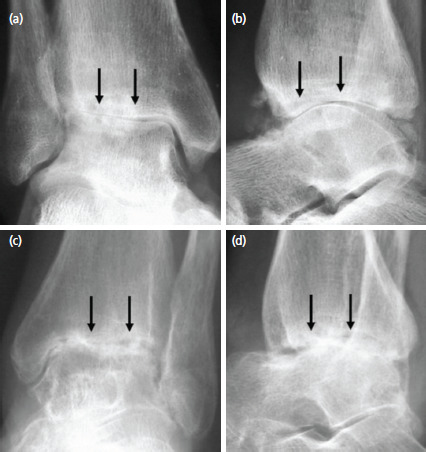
(a) Anteroposterior and (b) lateral radiographs of the right ankle joint of the second case with (c, d) corresponding views of the left ankle joint of the third case. Arrows showing advanced arthritic changes.
